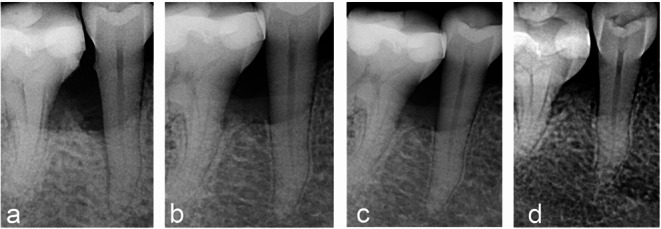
Treatment of an intrabony defect at a lower jaw premolar with A-PRF+. a preoperative radiograph. b 6 months after surgery partial fill of the intrabony component. c at 1 year defect fill is clearly visible. d at 5 years complete defect fill and stability
